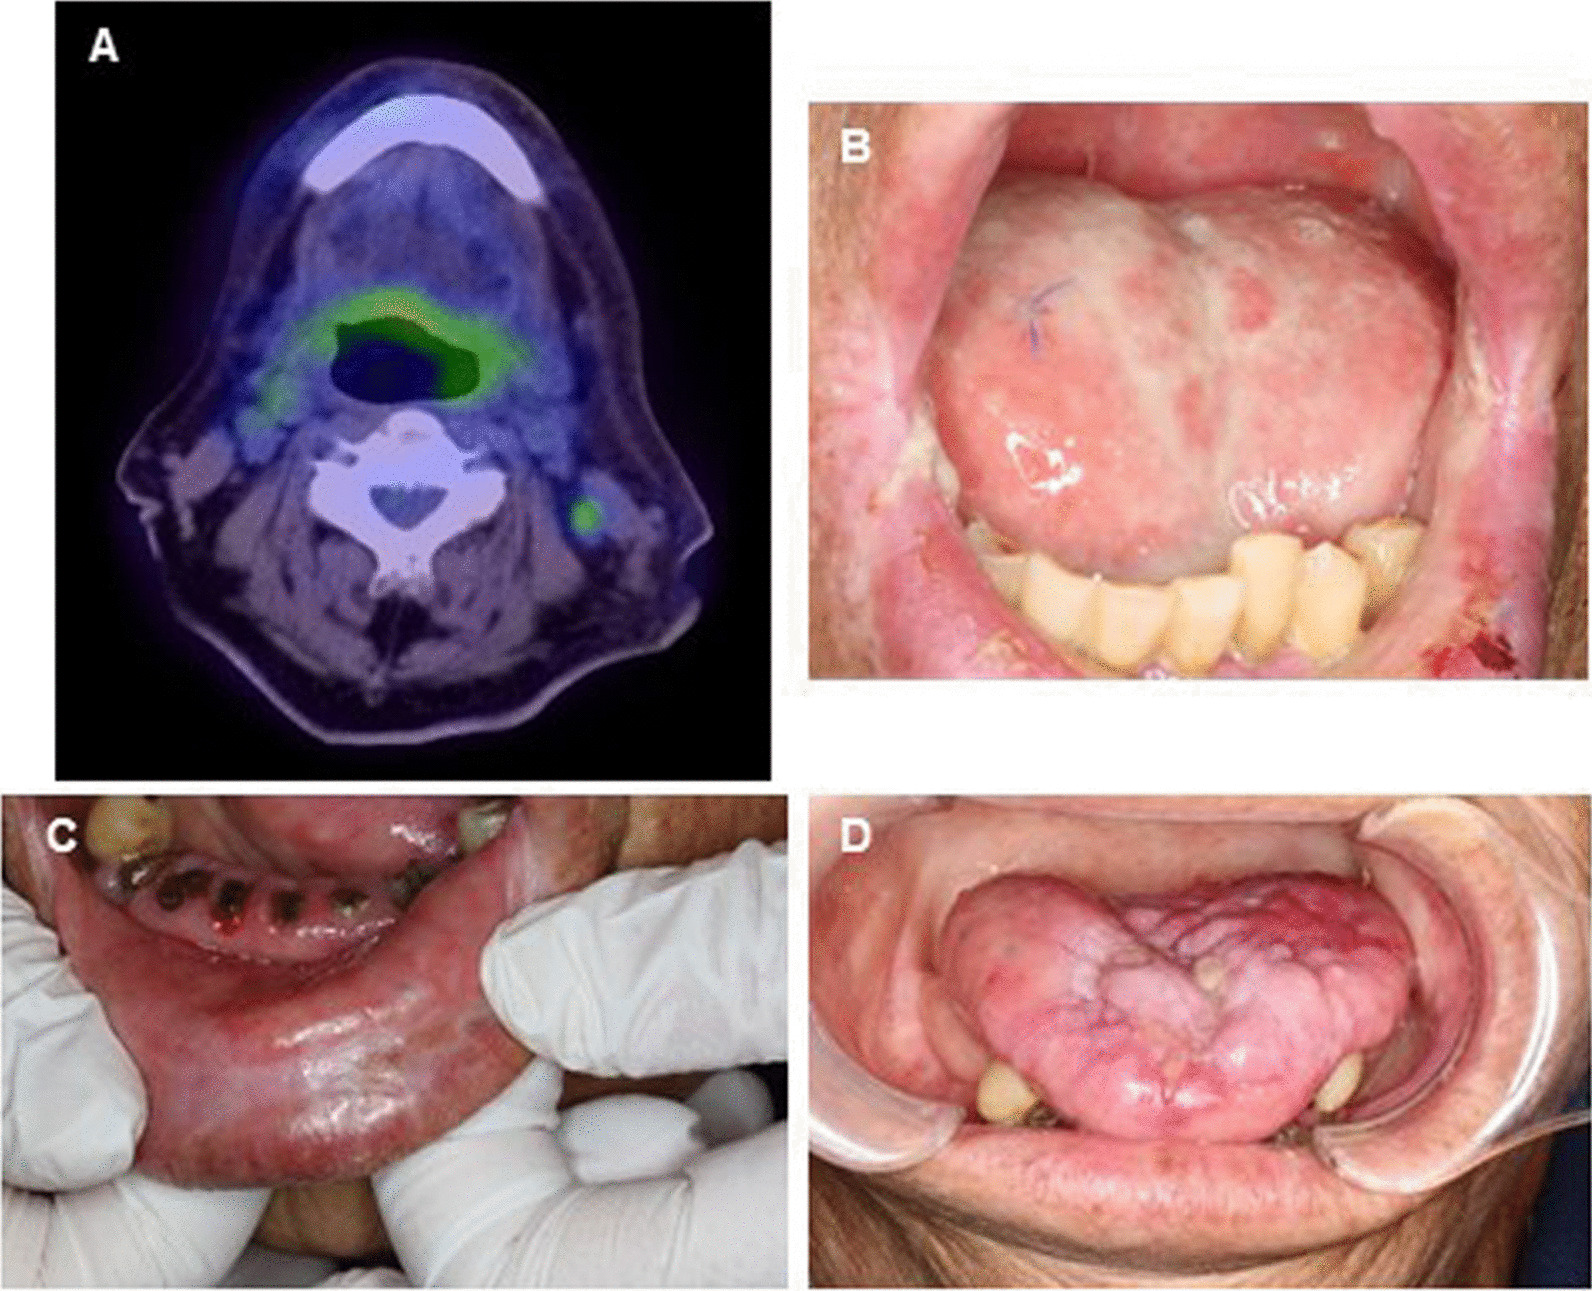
Representative images of synchronous multifocal oral squamous cell carcinomas following completion of treatment. Result of PET/CT one month after the treatment (A), local finding one month after the treatment (B), and local finding 3 years after the treatment (C, D)
